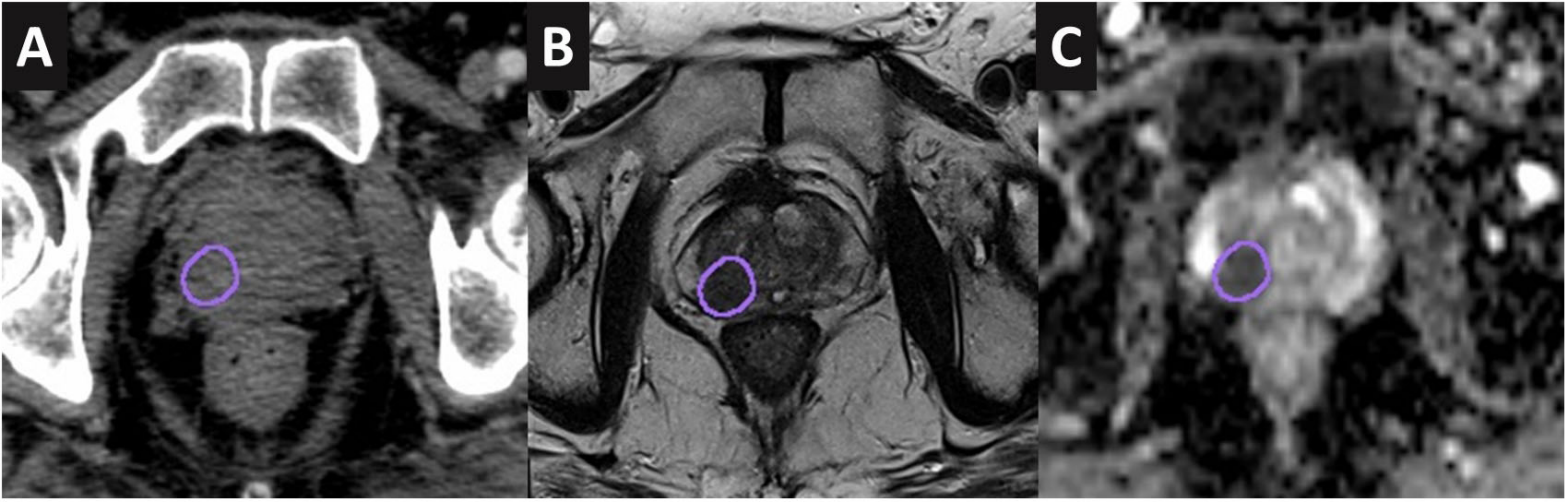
DIL contoured onto the CT scan (A), T2W MRI (B), and apparent diffusion coefficient map (C) of a case study.
